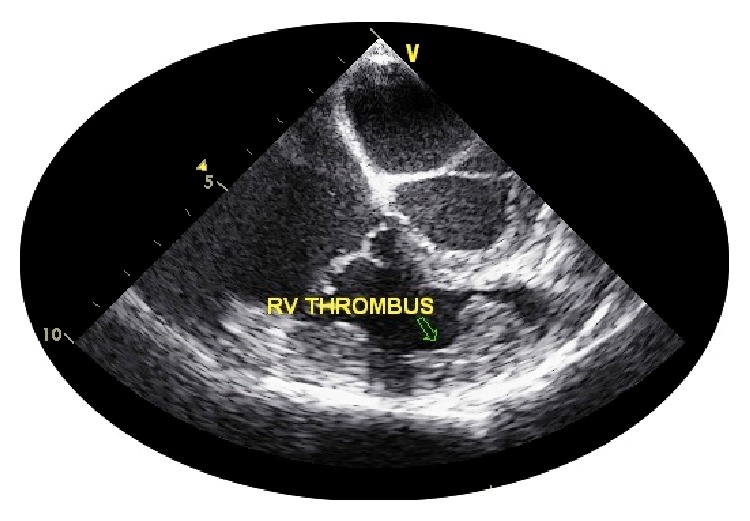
Transoesophageal Echocardiographic (TOE) image at midoesophagus (short axis) showing the right ventricular (RV) thrombus (arrow mark) along the free wall of RV, extending from just below tricuspid valve to the right ventricular outflow beneath the pulmonary valve.
